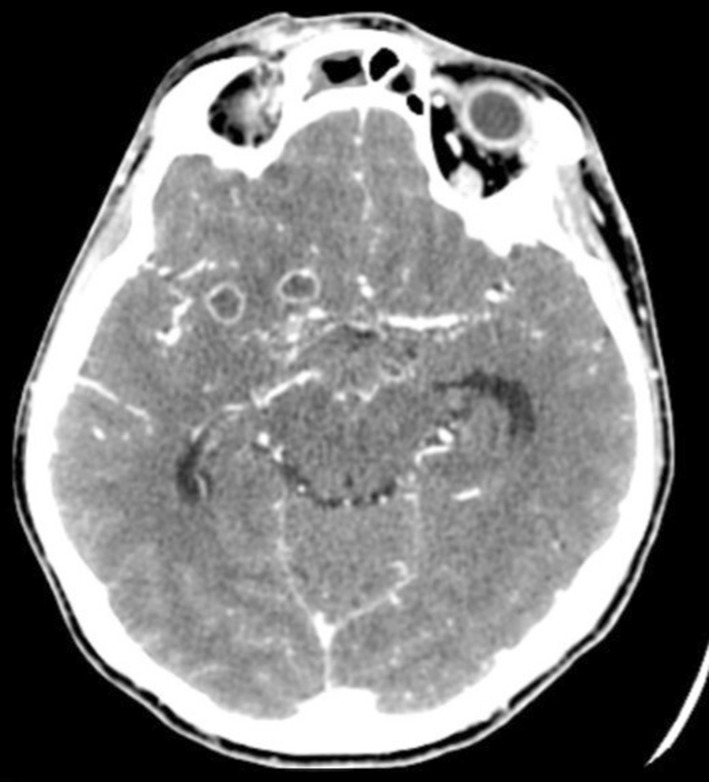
MRI of the cranium in a 28-year-old woman. Two small abscesses with diameters of 8 and 10 mm on the frontal–temporal border on the right side
